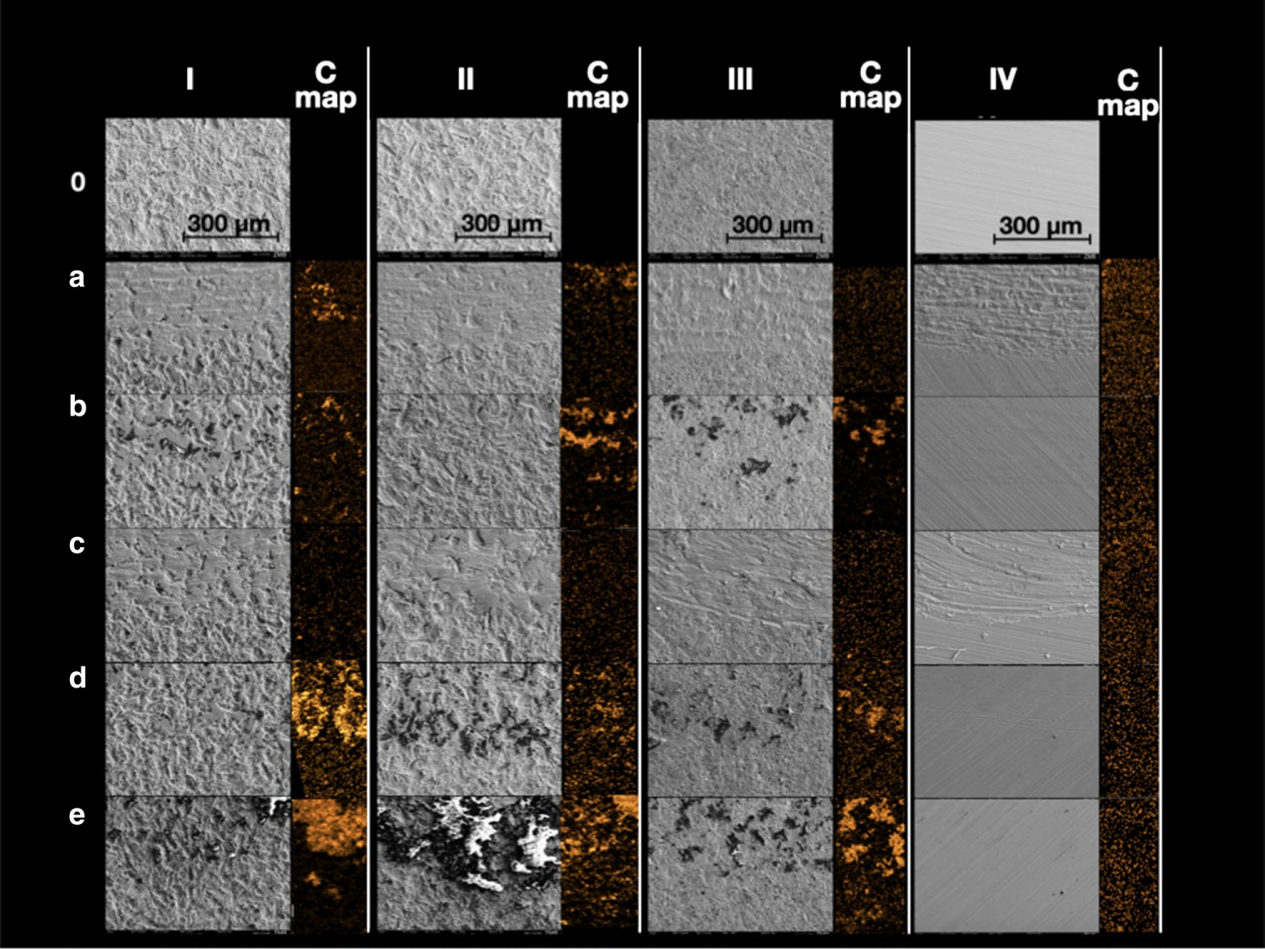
Titanium surfaces after ultrasonic instrumentation with different tips. Disc I—Inicell, Disc II—SLA, Disc III—non pretreated, Disc IV—machined. C map—EDX mapping for carbon distribution on the treated (upper half) and untreated (lower half) surface areas. 0—non instrumented surface, a steel, b PEEK, c titanium, d carbon, e resin
